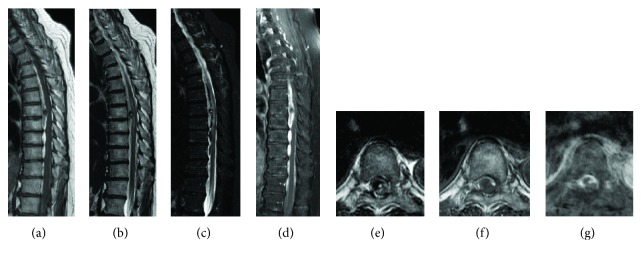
Sagittal (T1-weighted (a), T2-weighted (b), STIR (c), and contrast-enhanced (d)) and axial (T1-weighted (e), T2-weighted (f), and contrast-enhanced (g)) magnetic resonance imaging revealed a large subdural hematoma extending from T2 to L3 and compressing the spinal cord from the ventral side at 7 days after onset.
